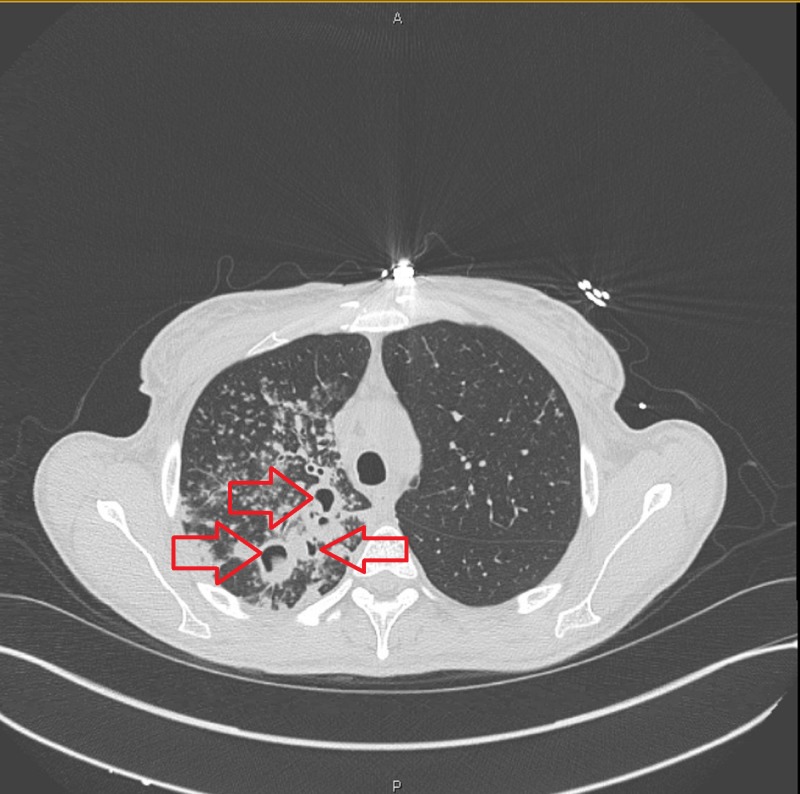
Chest computed tomography of the patient with tuberculosis. Multiple cavities surrounded by patches of consolidation with some traction bronchiectasis.
